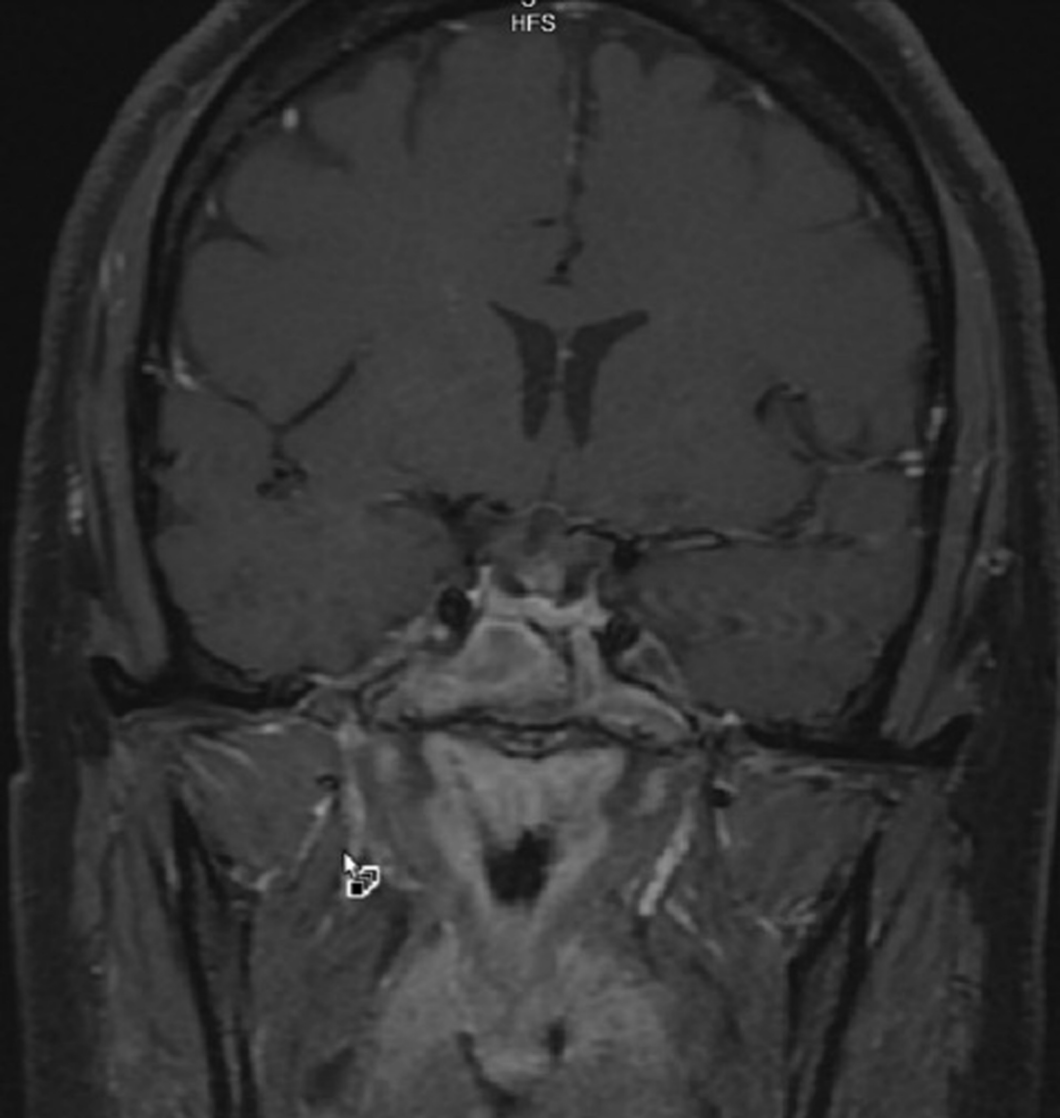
Coronal T1 (Post-operative) Post-operative brain MRI approximately 8 weeks after surgical resection of the lesion.
